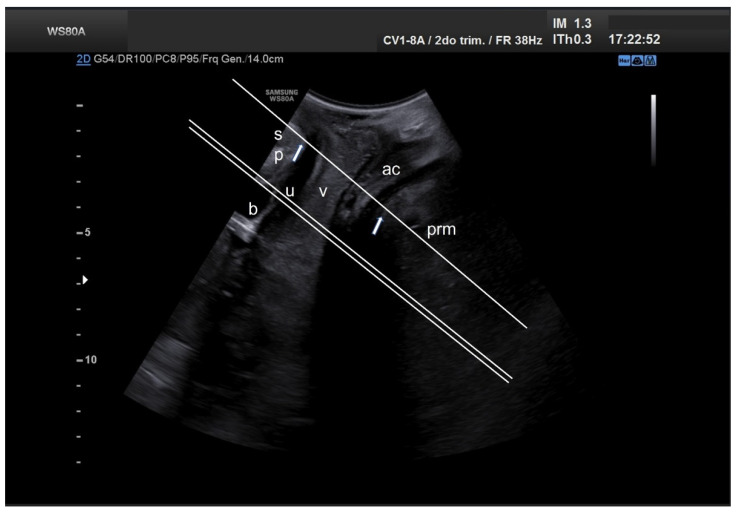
Mid-sagittal translabial two-dimensional pelvic floor ultrasound, showing the location of planes used for determining hiatal diameters and areas (single line) as well as pubovisceral muscle thickness and area (double line). ac, anal canal; b, bladder; prm, puborectalis muscle; sp, pubic symphysis; u, urethra; v, vagina.
